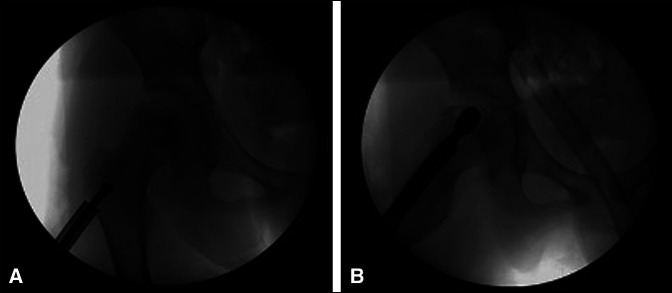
A, Radiograph showing a percutaneous expandable reamer system (X-REAM, Wright Medical) inserted through the cannula in a “closed position.” B, Radiograph showing the reamer within the lesion in the “open” position.
